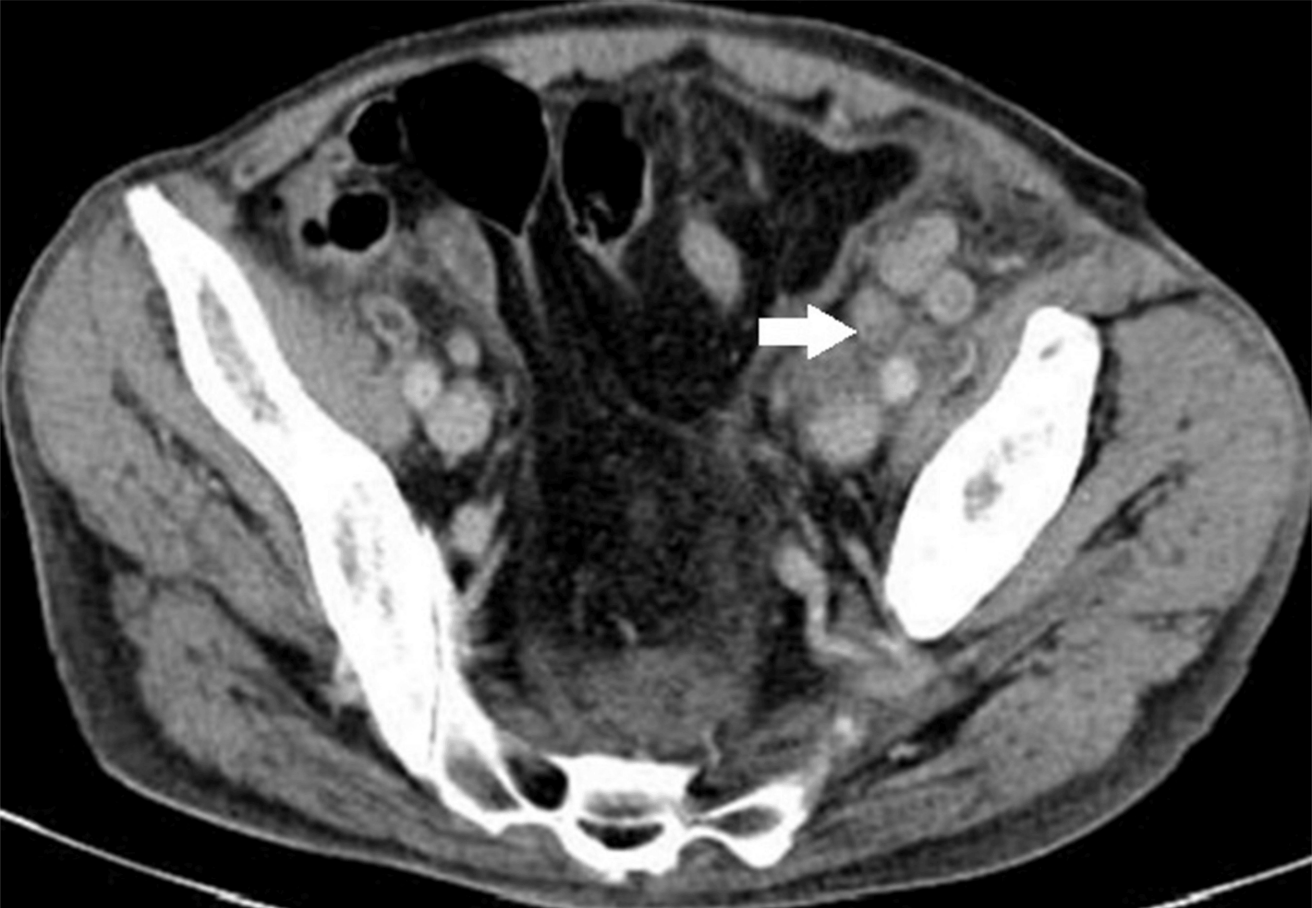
Abdominal CT showing multiple adenopathies, specifically iliac lymphadenopathies, the largest measuring 2 cm in short axis.
